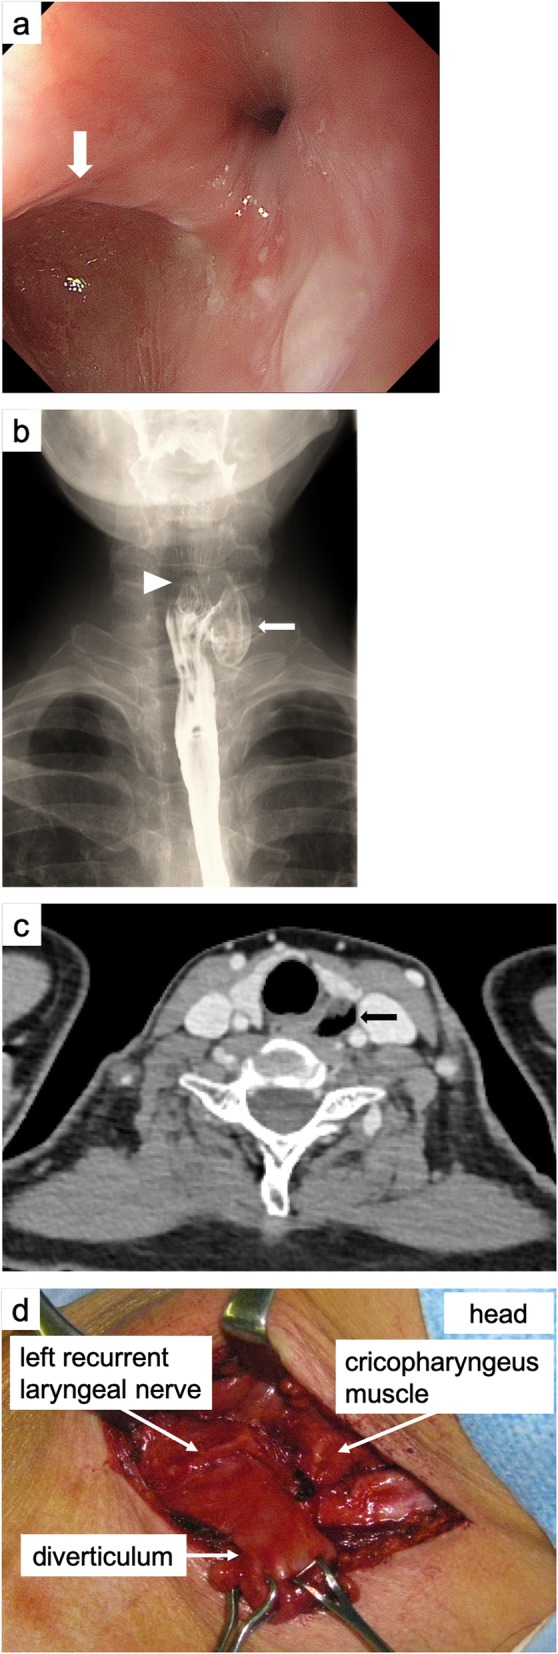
Preoperative and intraoperative findings of Case 1. a An esophagogastroduodenoscopy showed a diverticulum just under the esophageal orifice (white arrow). b An esophagogram showed a 2.5 cm-diameter diverticulum in the left side of the cervical esophagus (white arrow) and showed a cricopharyngeal bar presenting the cricopharyngeus muscle above the diverticulum (white arrowhead). c A cervical computed tomography showed an air-filled diverticulum arising from the left side of the cervical esophagus (black arrow). d Intraoperative findings. The diverticulum originated from the lateral wall of the cervical esophagus below the cricopharyngeus muscle, and the left recurrent laryngeal nerve lied very close to the base of the diverticulum.
